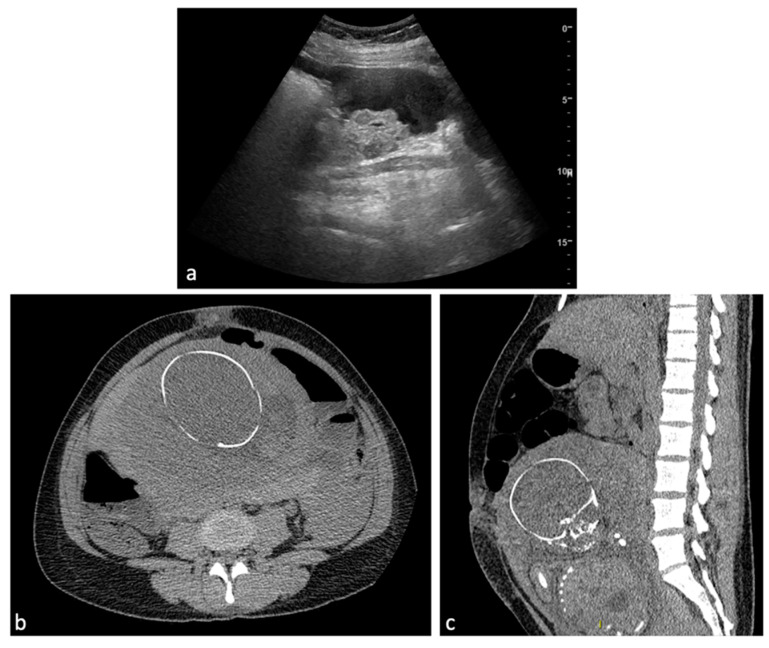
Uterine rupture. A 39-year-old female patient with a history of laparoscopic myomectomy who was pregnant at 29 weeks presented to the emergency department with abdominal pain and vaginal bleeding. Transabdominal ultrasound demonstrated hemoperitoneum (a) and a live fetus. CT scan without contrast medium (axial, b; sagittal, c) was performed urgently with confirmation of abundant hemoperitoneum. An urgent caesarean section was performed, with evidence of uterine rupture at the posterior wall in the presence of placenta accreta. Hysterectomy was performed.
